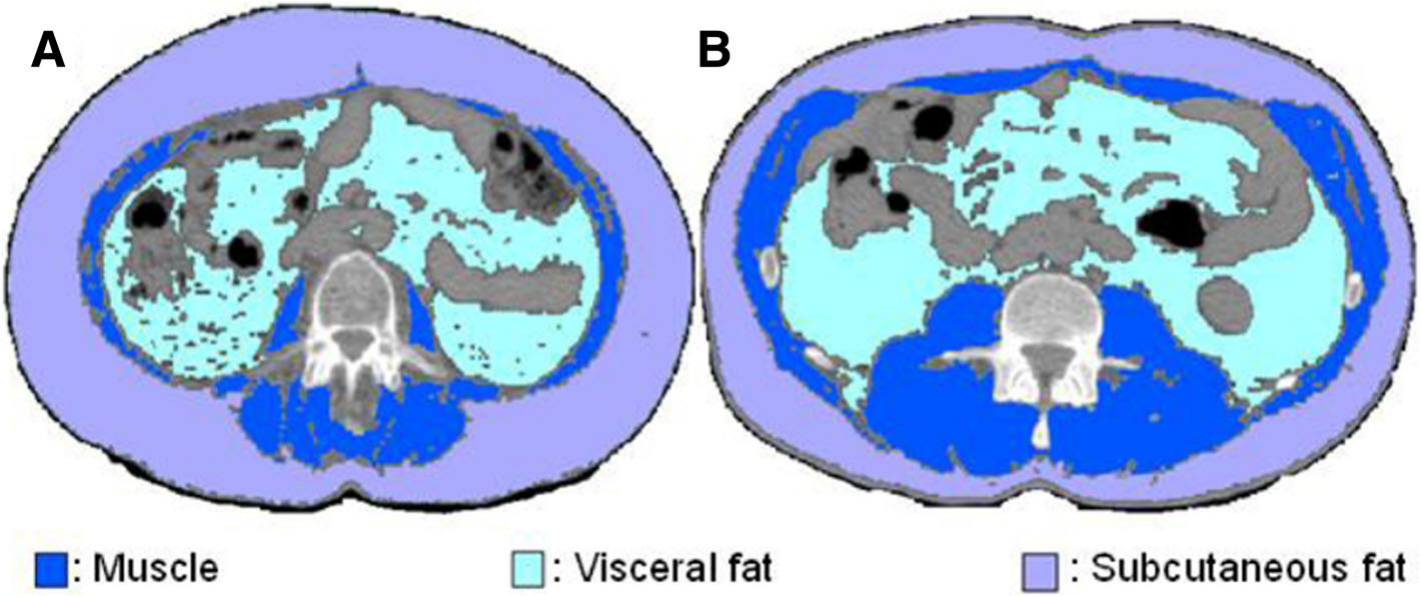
CT images of the third lumbar vertebra region in a sarcopenic (a) and a non-sarcopenic (b) patient
